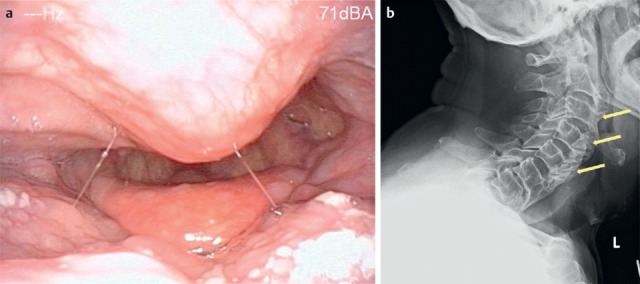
Forestier’s disease in a 75-year-old female patient. Dysphagia because of prominent hyperostosis of the cervical spine: a – endoscopically, a clear midline protrusion of the posterior pharyngeal wall is visible with relation to the base of tongue and epiglottis; b – lateral radiography of the cervical spine of the same patient with visible bony attachments to the vertebrae and ligamentous structures (C2–6, see arrows).
